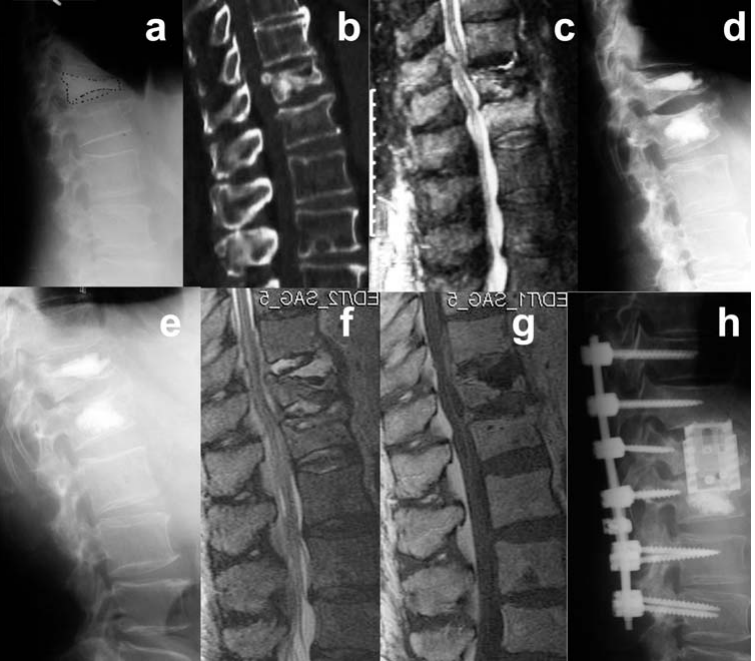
This 68-year old man with corticoid-induced secondary osteoporosis and multiple co-morbidity fell at home and presented with osteoporotic fractures at T12 and L1 (a, b). The MRI confirmed fresh fractures and revealed a spinal stenosis at T12/L1 (c). Since non-surgical therapy was not successful, neurological deficits were not prevalent, kyphoplasty at T12 and L1 was performed as a minimal intervention (d). Postoperatively the patient was mobilised and left the hospital 4 days after kyphoplasty. Two weeks later the patient was admitted to our emergency care unit with incomplete paraplegia sub T8. Laboratory diagostics revealed highly elevated leukocytes and C-reactive protein. Plain radiographs showed a thin radiolucency around the cement core on T12 (e). The MRI confirmed the suspected spondylitis and found additionally an epidural abscess (f, g). Therefore posterior decompression with instrumentation from T10 to L3 was performed and anterior corporectomy of T12 with complete cement removal and implantation of an expandable titanium-cage and bone graft was performed (h). An incomplete paraplegia sub L2 remained.
